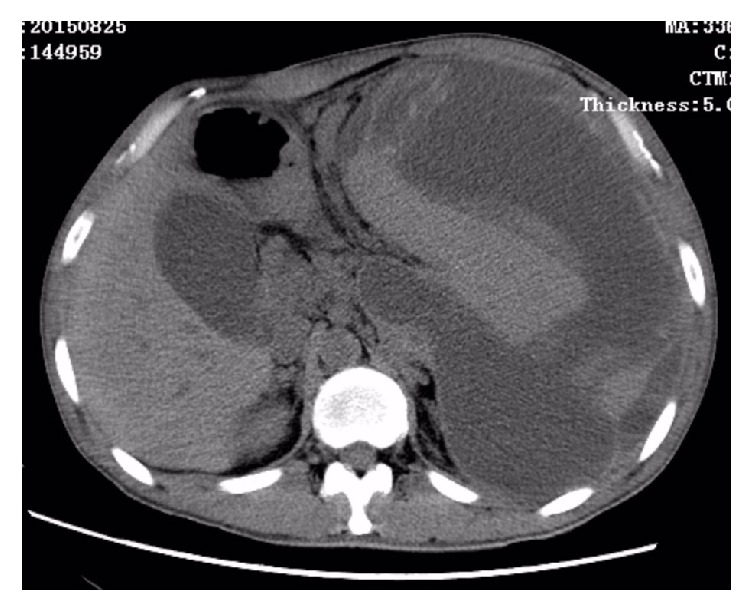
CT scan showing a large subcapsular splenic hematoma (16.0 × 16.0 × 7.6 cm) with a large pancreatic pseudocyst (13.5 × 10.0 × 8.0 cm).
